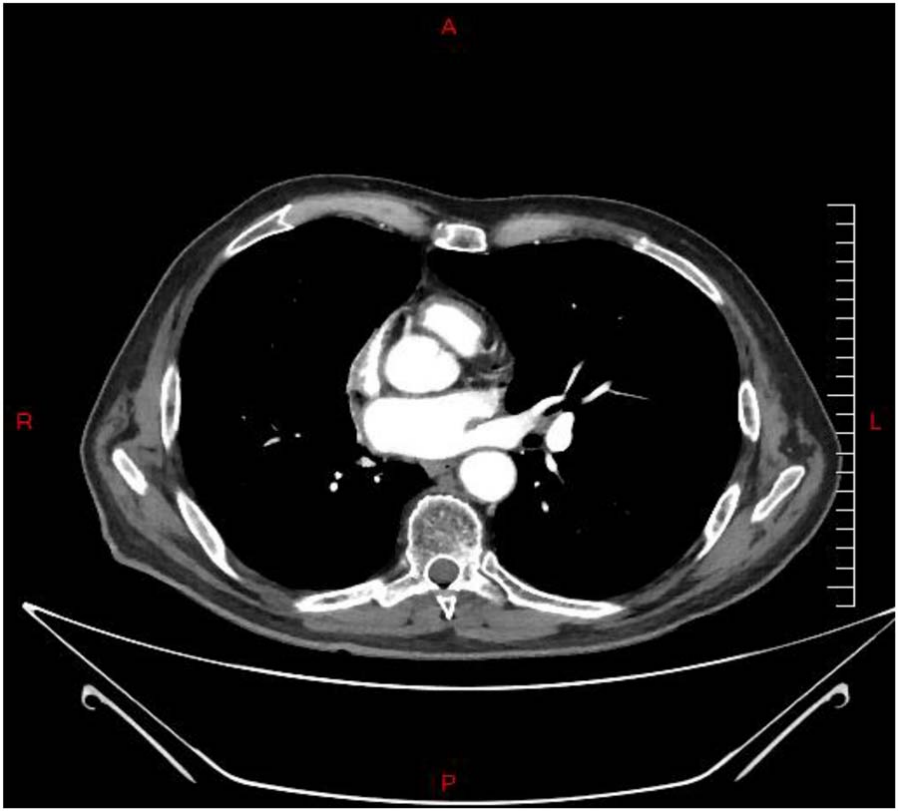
Axial contrast CT chest revealed normal finding with no evidence of tumour activity.
